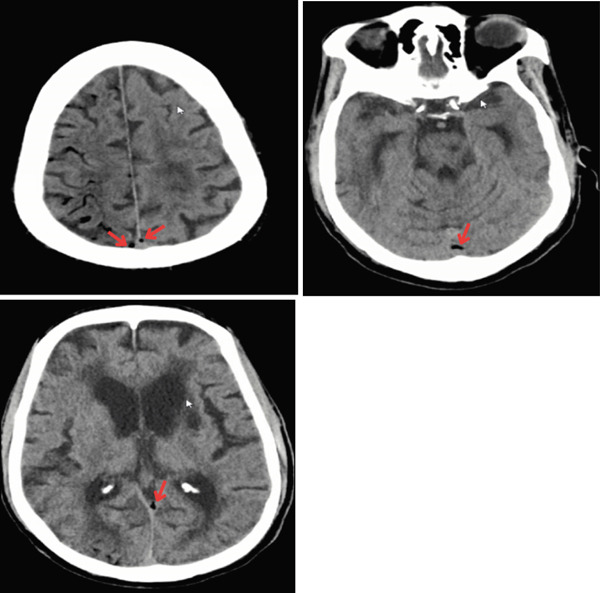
CT scan of the brain showing air embolism in the cerebral venous system, marked by red arrows.
